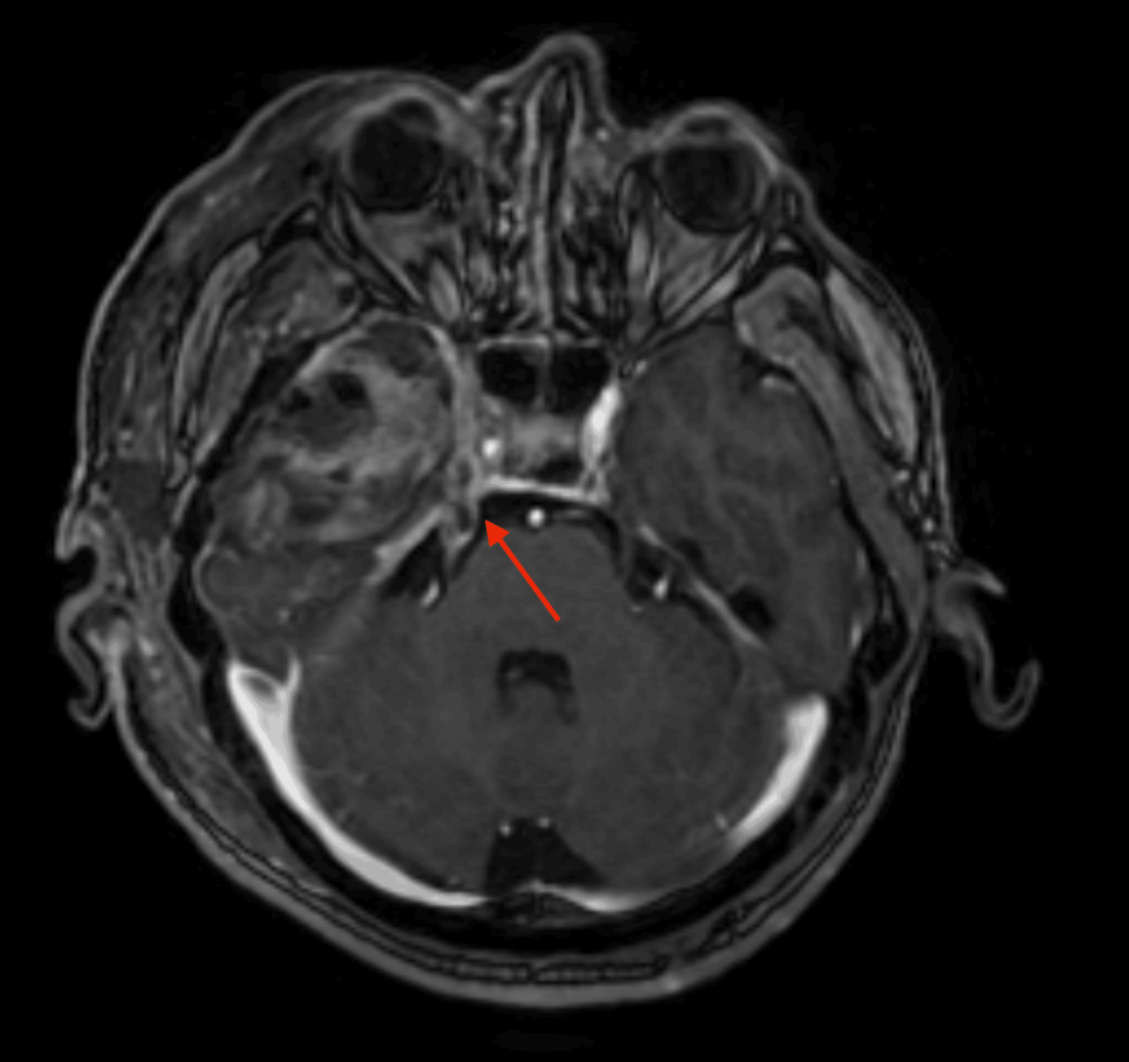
MRI of the brain MRI of the brain shows extensive perineural spread of the tumor through the third division of the trigeminal nerve (red arrow).
